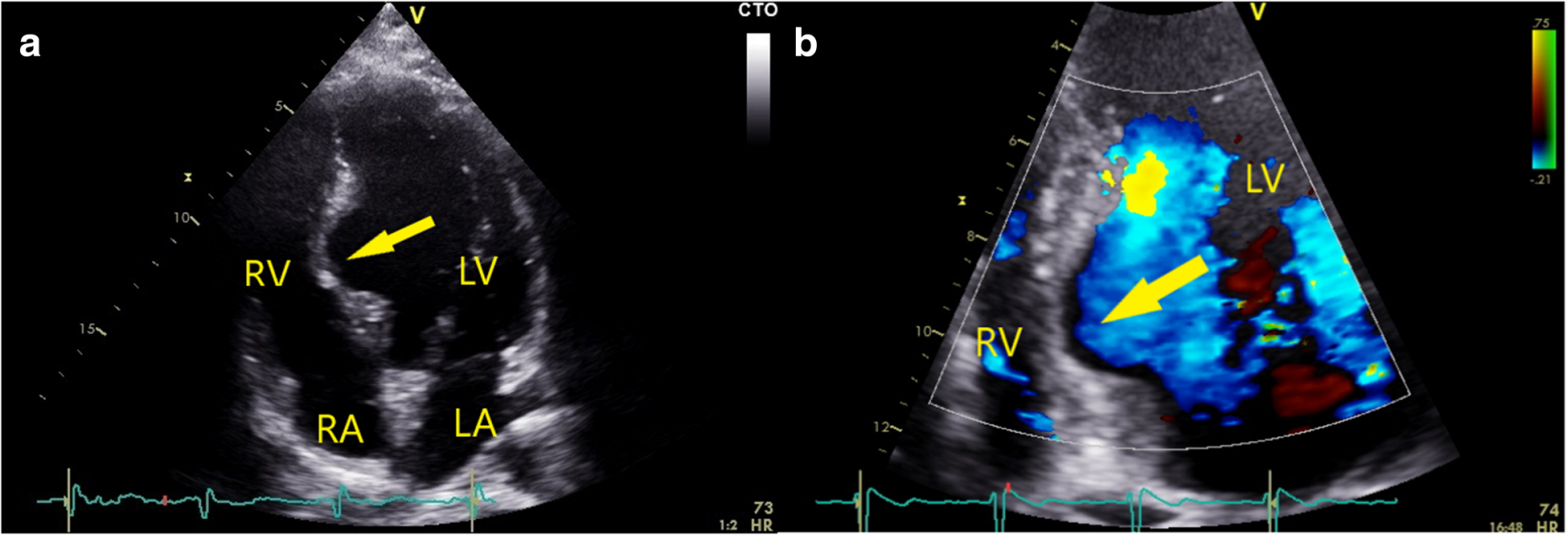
2D transthoracic echocardiography, apical 4-chamber view of the interventricular septum 6 months after the surgical procedure; (a) the arrow points to the residual aneurysm of the basal part of the intraventricular septum. b zoomed-in view of the interventricular septum with color flow Doppler showing no signs of interventricular shunt. (LA = left atrium, LV = left ventricle, RA = right atrium, RV = right ventricle)
